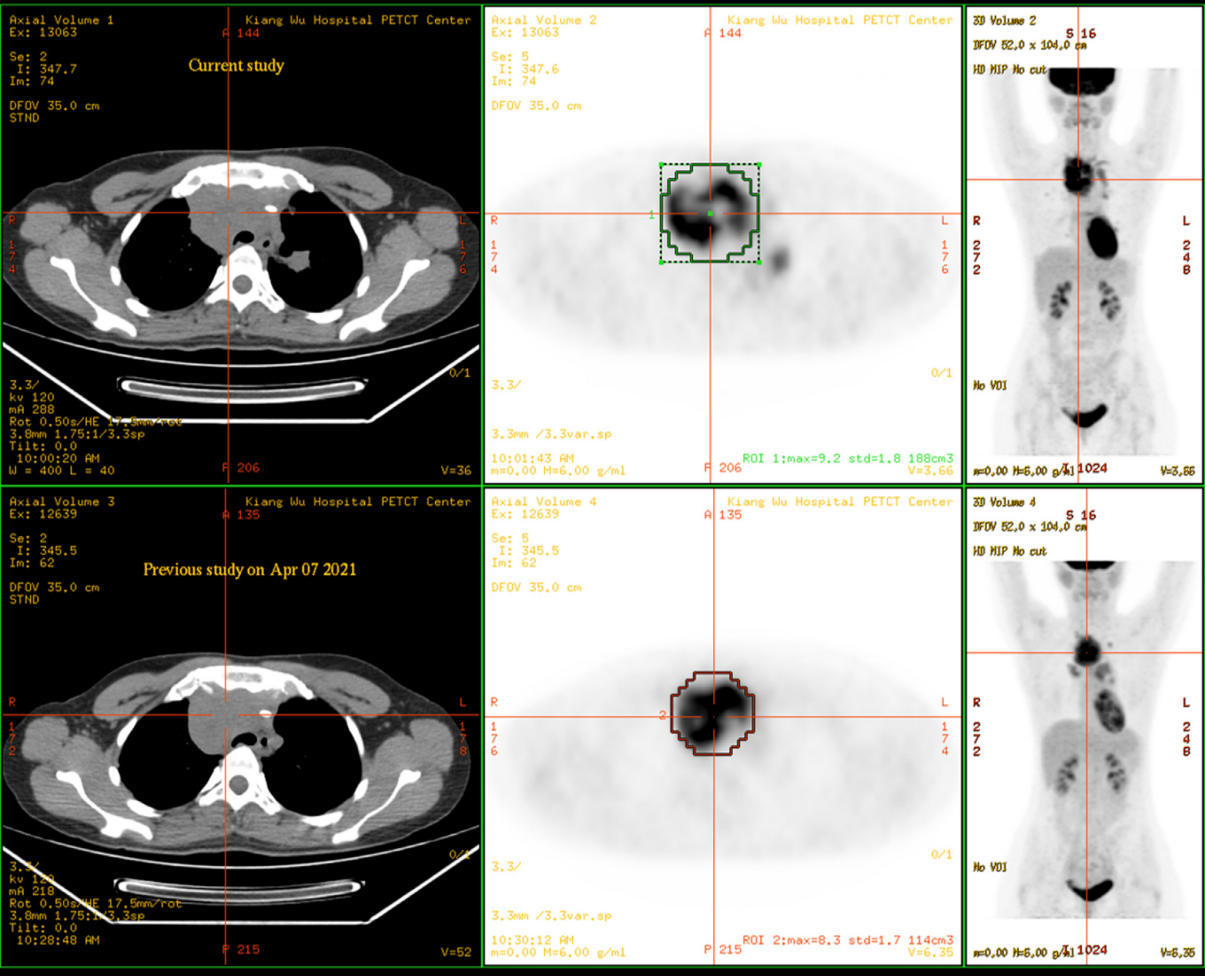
Progressive disease was identified after re-chemotherapy and target therapy. The work-up ^18^F-FDG PET/CT on June 26, 2021 showed worsening of the recurrent tumor with hypermetabolism in the mediastinum after re-chemotherapy and target therapy.
